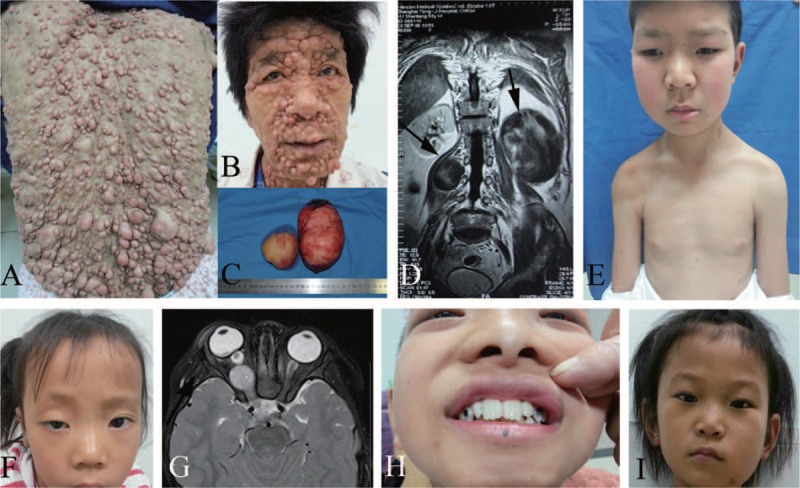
Clinical manifestations of the patients. Gross generalized cutaneous neurofibromas (CN) on the back (A) and face (B) of patient 28. (C) Two malignant peripheral nerve sheath tumors (arrows) from patient 18 with 7-cm and 9.5-cm diameters. (D) Trunk axial MRI shows 2 low-signal-intensity lesions in T1-weight imaging. (E) Macrocephaly in patient 27. (F) Optic gliomas (OG) in patient 17. (G) Orbital axial CT of patient 17 shows OG of her right eye (arrow). (H) Dental caries in patient 26. (I) Facial dysmorphism in patient 2. CT = computed tomography, MRI = magnetic resonance imaging.
